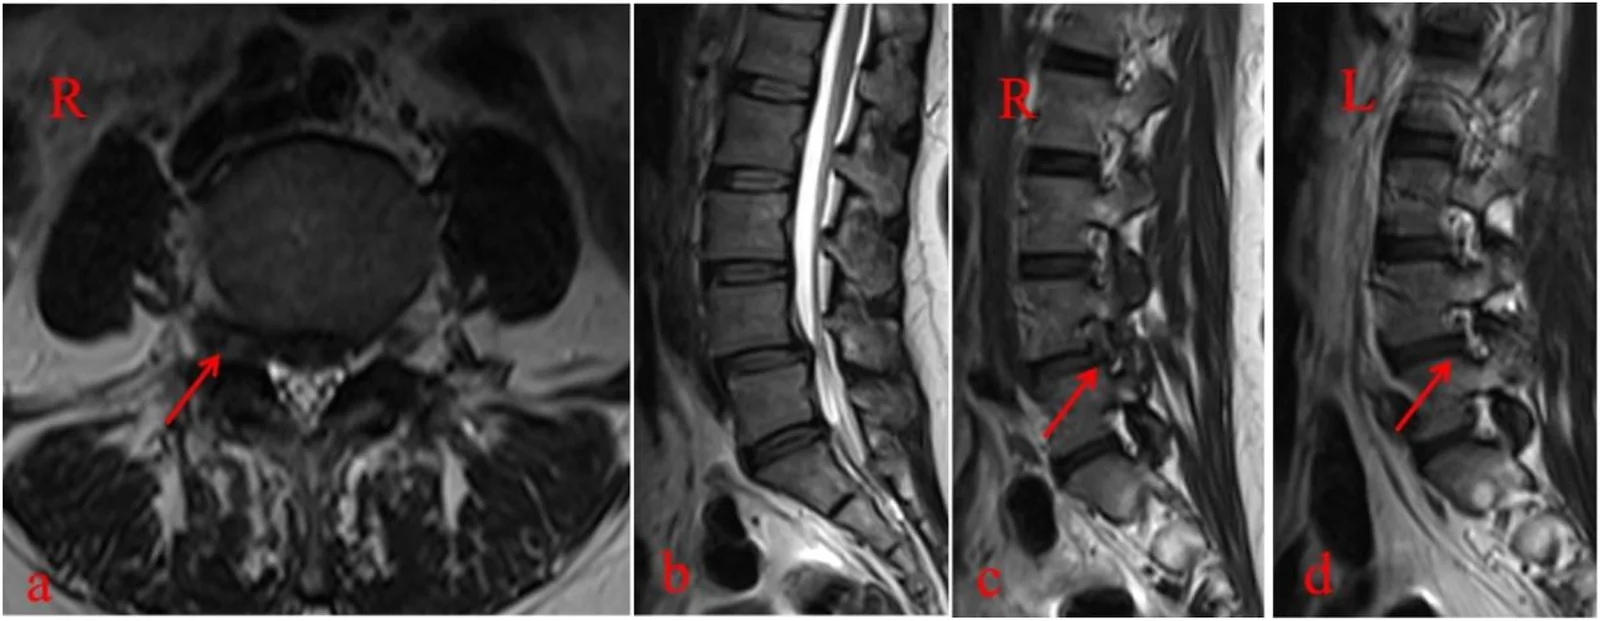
A preoperative MRI revealed L4/5 disc herniation (a,b) and right foraminal stenosis secondary to a sequestered disc fragment (a,c), with the left foramen appearing nearly normal (d).
